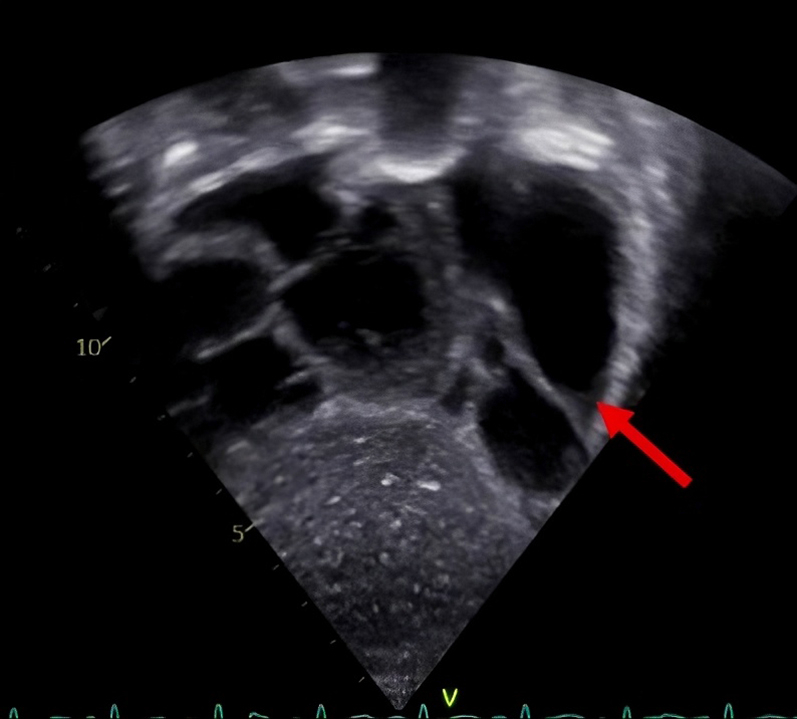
Transthoracic Echocardiogram A large cystic structure lateral to the left ventricle (red arrow) in a subcostal long-axis view.
